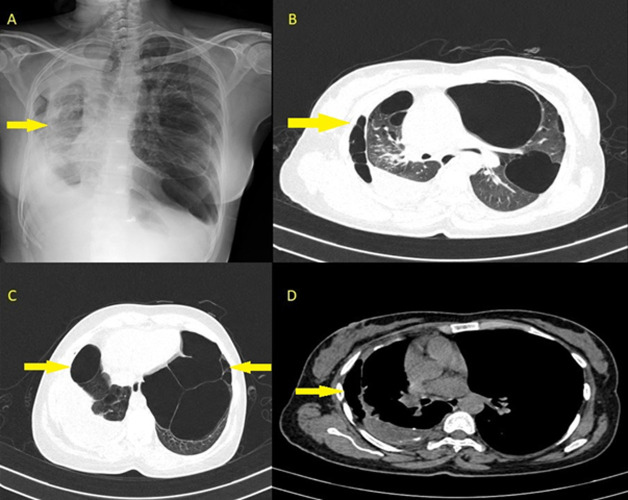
A) chest X-ray of the patient; high-resolution computed tomography of the patient’s thorax showing: B) pleural effusion with multiple air foci within the pleura; C) multiple bullae; D) thickened visceral and parietal pleura
